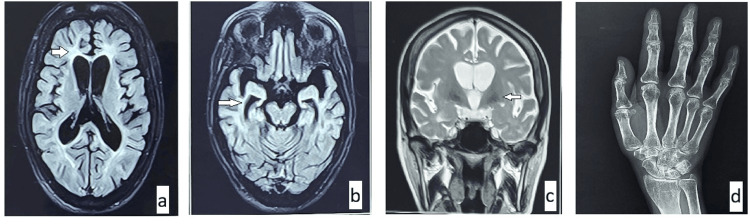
MRI brain and X-ray findings. (a) and (b) FLAIR images showing periventricular white matter hyperintensity and diffuse cortical atrophy. (c) T2 image showing bilateral globus pallidus calcification. (d) X-ray of the right hand demonstrating periarticular osteopenia. FLAIR: fluid-attenuated inversion recovery.
